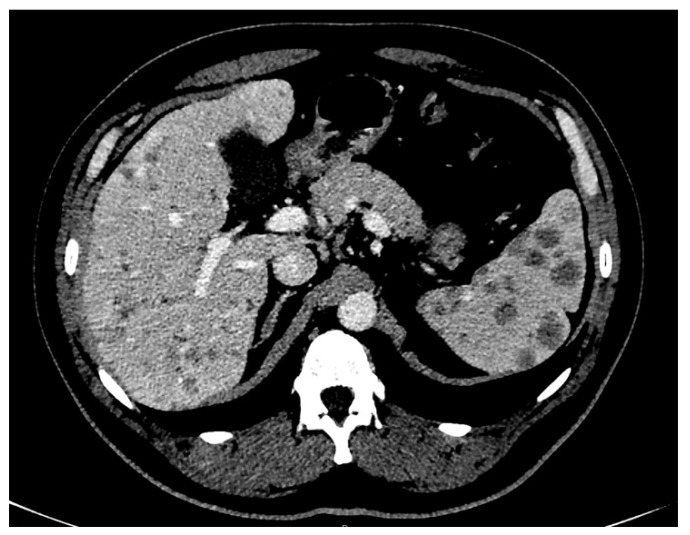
Abdominal contrast-enhanced computed tomography, in portal phase, showed multiple nodules ranging from 1.0 or 2.0 mm to 0.75 cm in diameter diffusely distributed in the liver, and ranging from 1.0 mm to 2.0 cm in the spleen.
